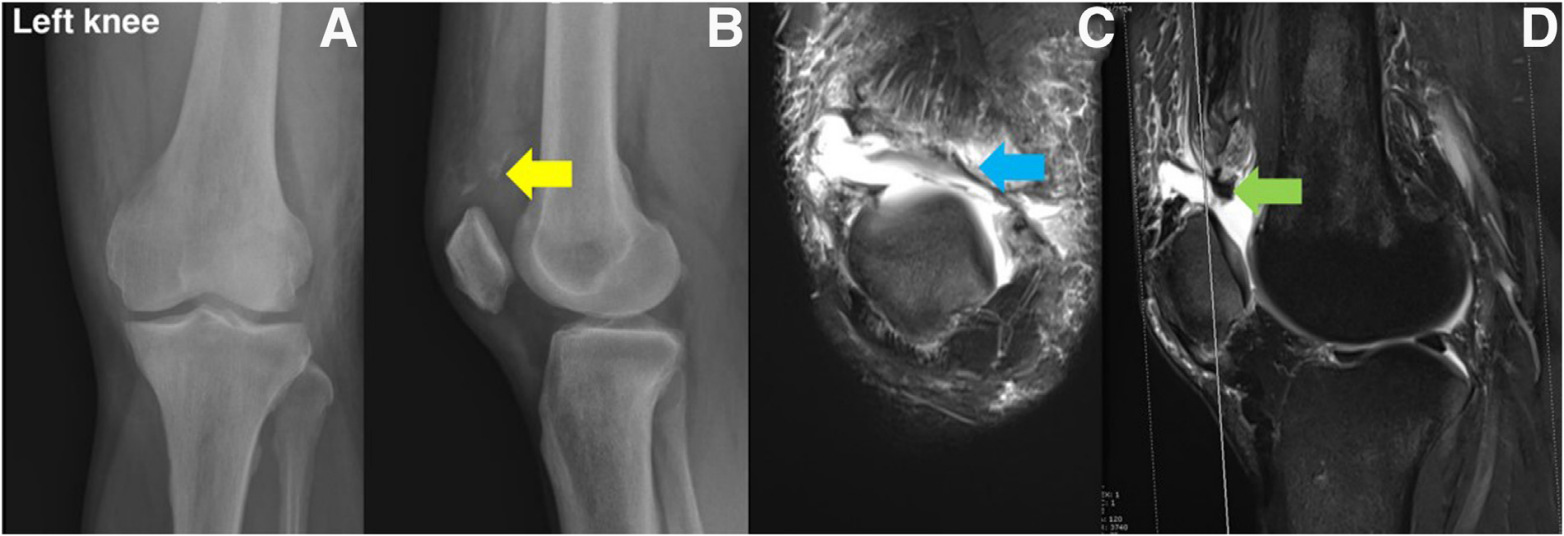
Radiographs of the left knee show downward displacement of the left patella with calcified deposits in the quadriceps tendon (yellow arrow): anteroposterior view (A) and lateral view (B). Magnetic resonance imaging of the left knee (T2 fat suppression (T2FS) in coronal plane) shows a rupture at the proximal patellar bone–tendon junction with extension to the medial and lateral retinaculum (blue arrow) (C) and shows complete disruption at the distal quadriceps bone-tendon junction and avulsion of the quadriceps tendon from the superior pole of the patella (green arrow) (D).
